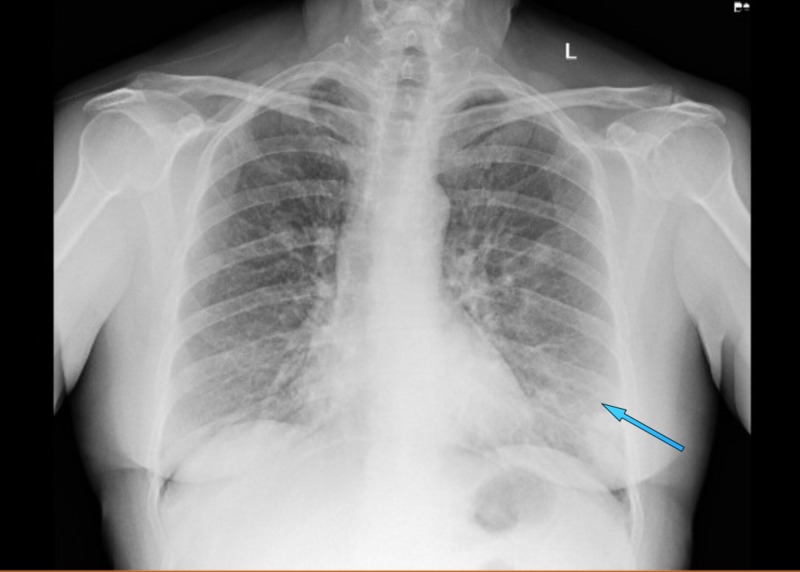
Initial anterior-posterior chest radiograph obtained on hospital admission showing a small left basilar airspace opacity (blue arrow)
